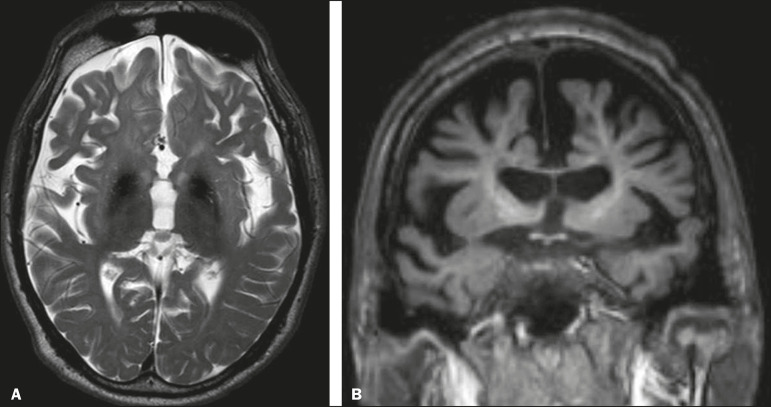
A 37-year-old male patient with progressive neurological deterioration. Axial T2-weighted and coronal T1-weighted MRI sequences (A and B, respectively) showing bilateral reduction in the volume of the encephalon beyond what would be expected for the age bracket, predominantly in the frontotemporal region, and preservation of the posterior cortical regions (A).
